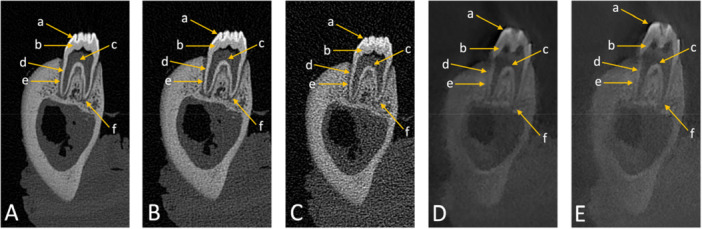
Coronal images of dental anatomy, depicting the first molar, visualized in (A) high‐dose photon‐counting detector computed tomography (PCD‐CT) reconstruction, (B) standard‐dose PCD‐CT reconstruction, and (C) low‐dose PCD‐CT reconstruction, alongside manufacturer‐specific cone‐beam computed tomography (CBCT) scans at (D) standard‐dose and (E) low‐dose protocols. Key structures are labeled as follows: a: enamel; b: dentine; c: pulp: d: root canals; e: periodontal space; f: apical foramen.
